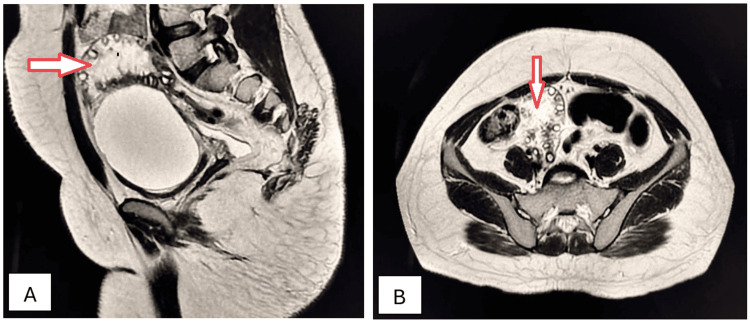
Enlarged right polycystic ovary (arrows). Sagittal (A) and axial (B) T2W MRI imaging shows an enlarged right ovary with small, sub-centimeter, peripheral follicles arranged in a necklace pattern with no dominant follicle and prominent, hyperintense central stroma, consistent with a polycystic ovary. T2W: T2-weighted
